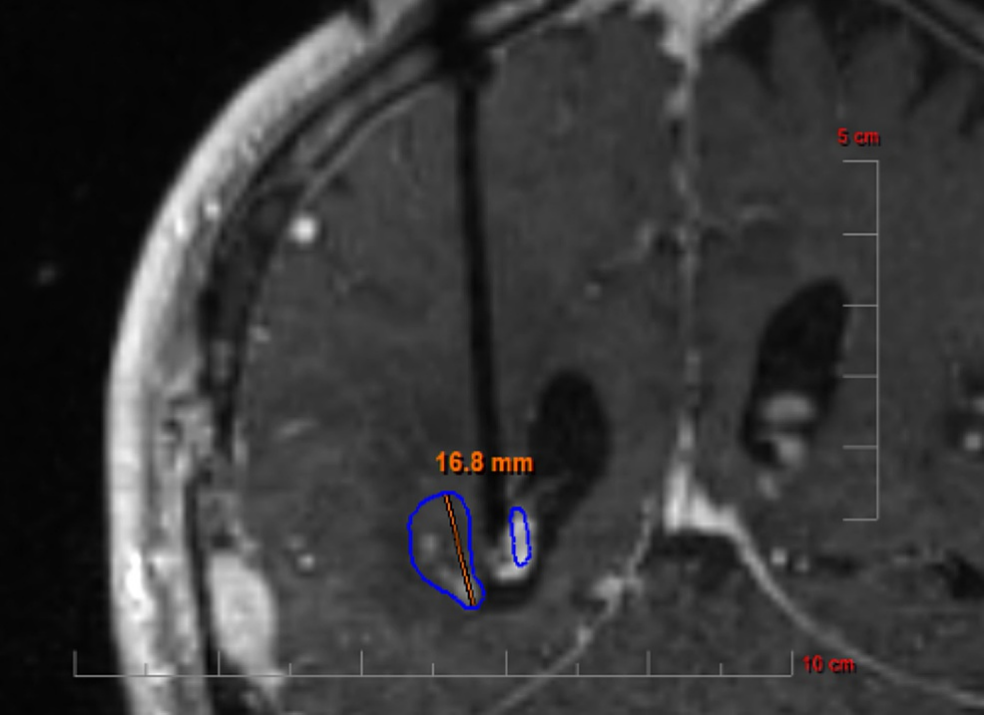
Contrast-enhanced MRI image of a patient undergoing laser-interstitial thermal therapy (LITT) for radiation necrosis. The blue line represents the line inside which biological equivalence of heating to 43˚ C at 10 minutes is delivered.
